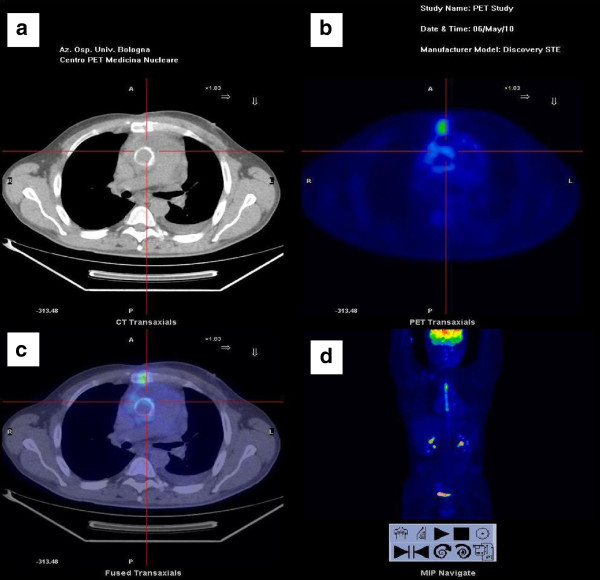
Case 1: the image below shows a focal uptake (SUVmax = 3.5) at anterior wall of mechanical aortic prostethic valve, consistent with an acute infection. a. CT. b. PET. c. Fusion image. d. Maximum intensity projection.
